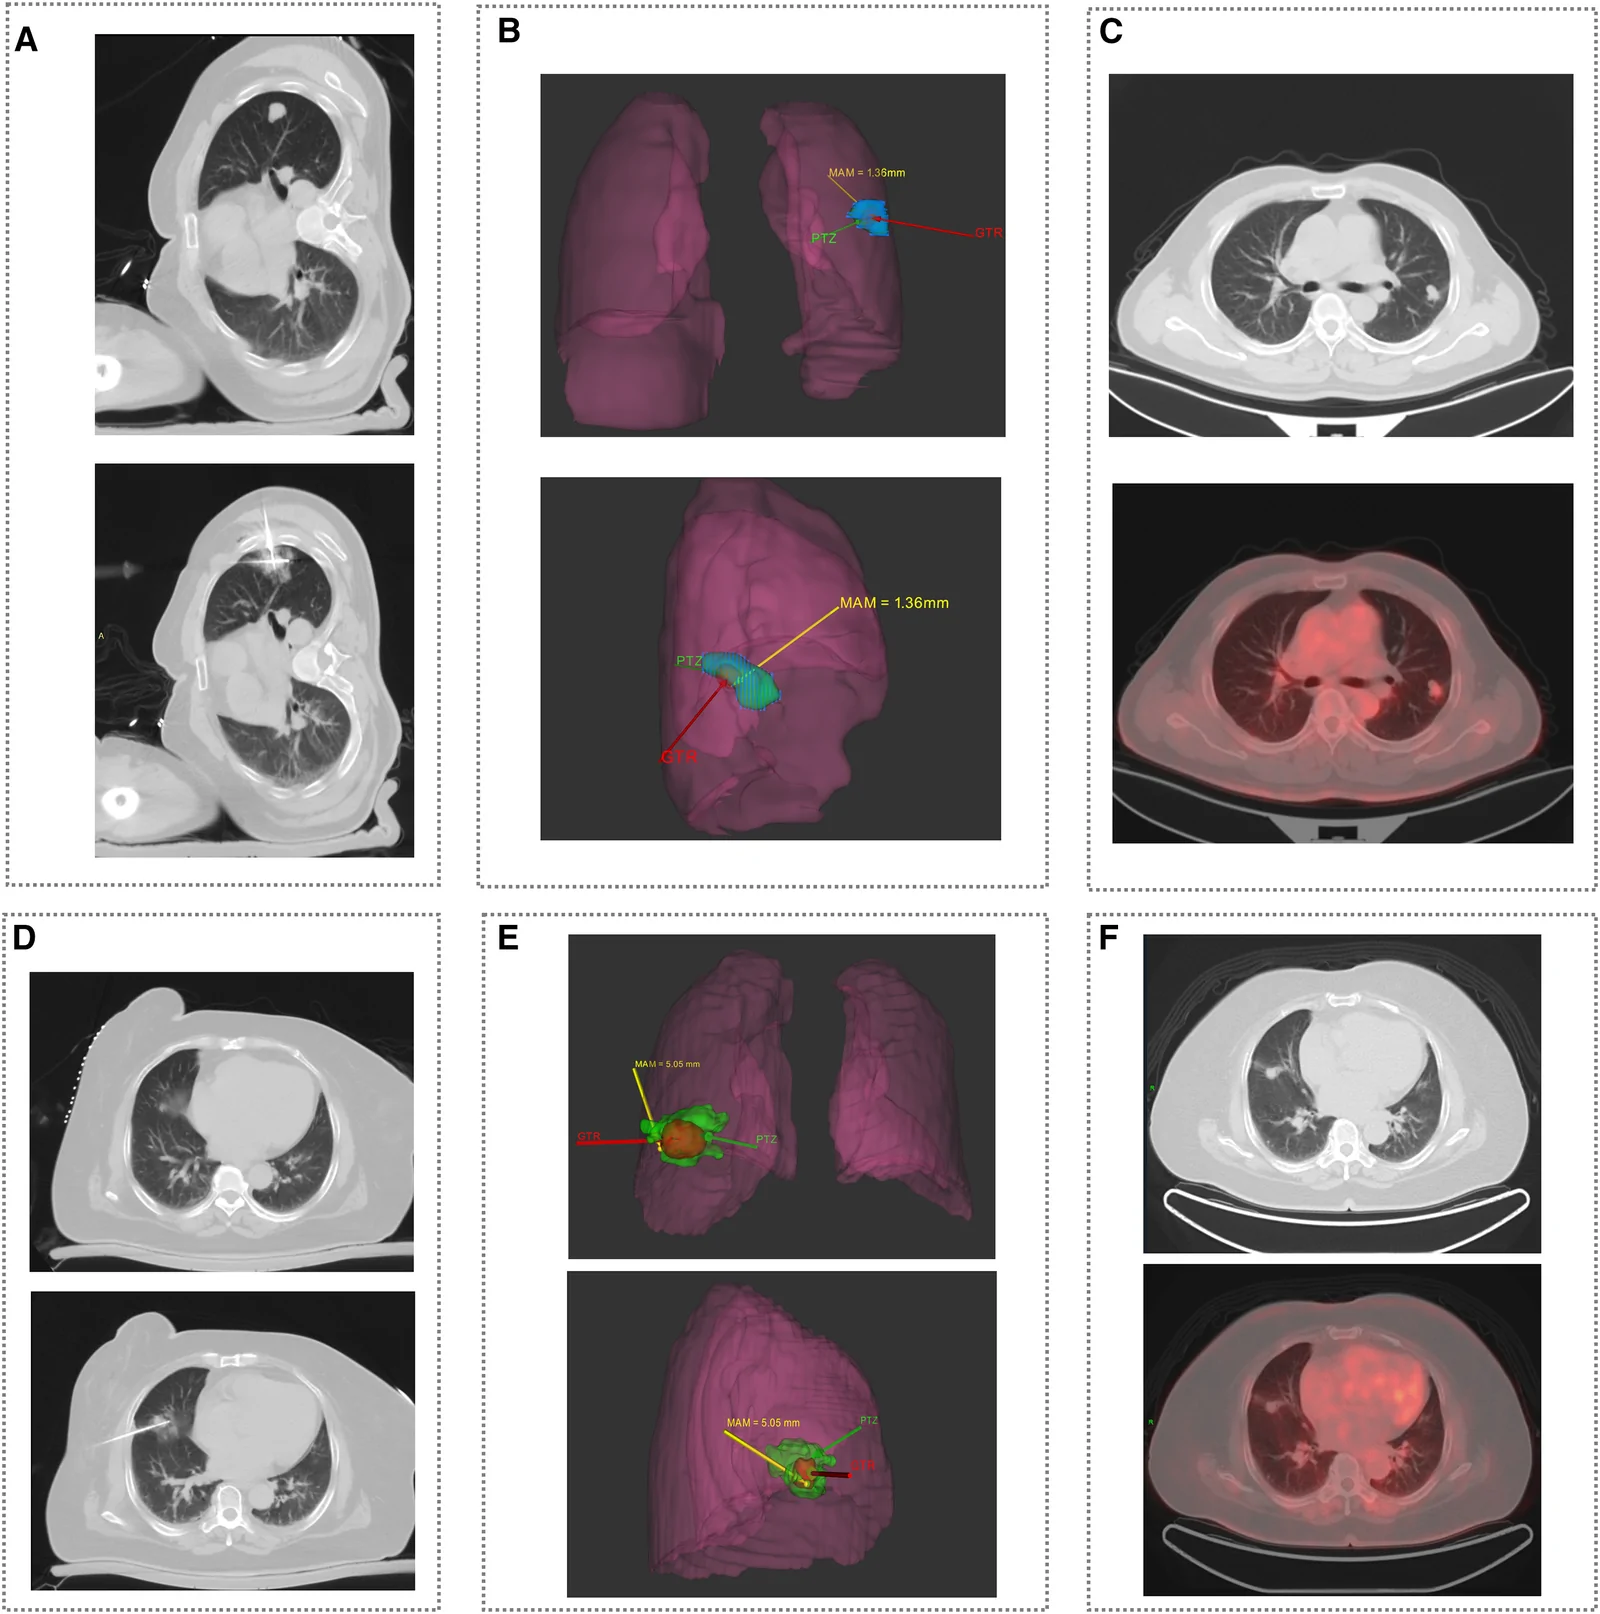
Representative three-dimensional MAM quantification in cases with insufficient and adequate margins (A) Pre-ablation and intraprocedural axial CT images from a representative case with an insufficient margin. (B) VTK-based 3D visualization of the spatial relationships among the post-treatment zone (PTZ, green contour), gross tumor region (GTR, red contour), and minimum ablation margin (MAM = 1.36 mm, yellow line). (C) Post-ablation PET/CT image shows focal residual metabolic activity in the case with an insufficient margin. (D) Pre-ablation and intraprocedural axial CT images from a representative case with an adequate margin. (E) Corresponding VTK-based 3D visualization shows complete tumor coverage with MAM ≥5 mm (representative MAM = 5.05 mm). (F) Post-ablation PET/CT image shows no focal residual metabolic activity in the case with an adequate margin.
